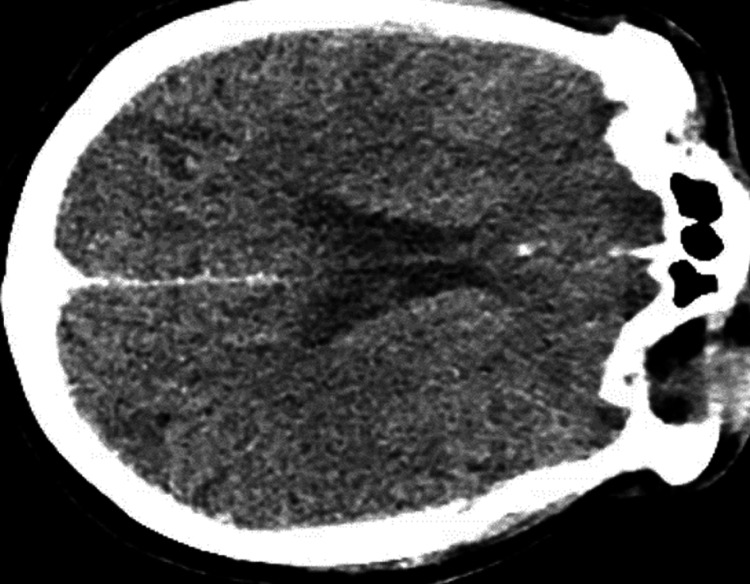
CT non-contrast of the head showing no evidence of an acute intracranial process CT: computed tomography
